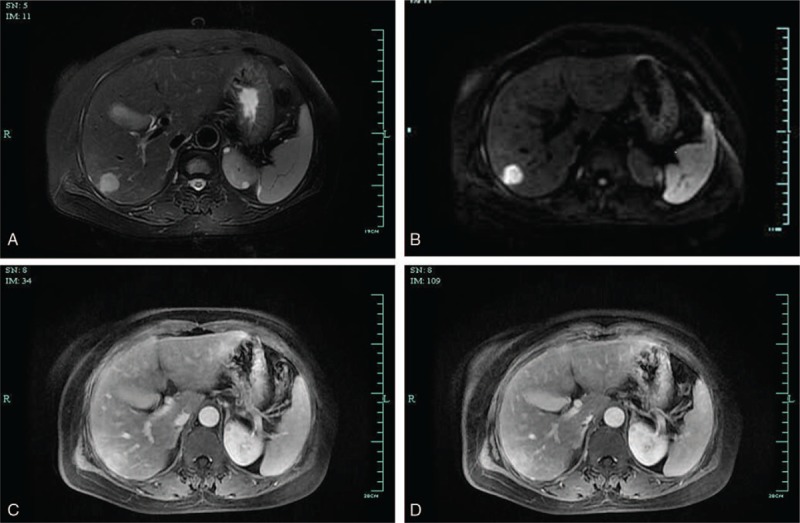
Abdominal magnetic resonance imaging (MRI) findings. The lesion was located in right liver measuring about 20 × 18 mm and showed low SI on T1WI, high signal intensity (SI) on T2-weighted imaging (T2WI) and high SI on diffusion-weighted imaging (DWI). The lesion showed enhancement on arterial phase and faded signal on portal and delayed phases. Hypervascular neoplasm was suspected by MRI. (A: T2WI; B: DWI; C: arterial phase; D: portal venous phase).
